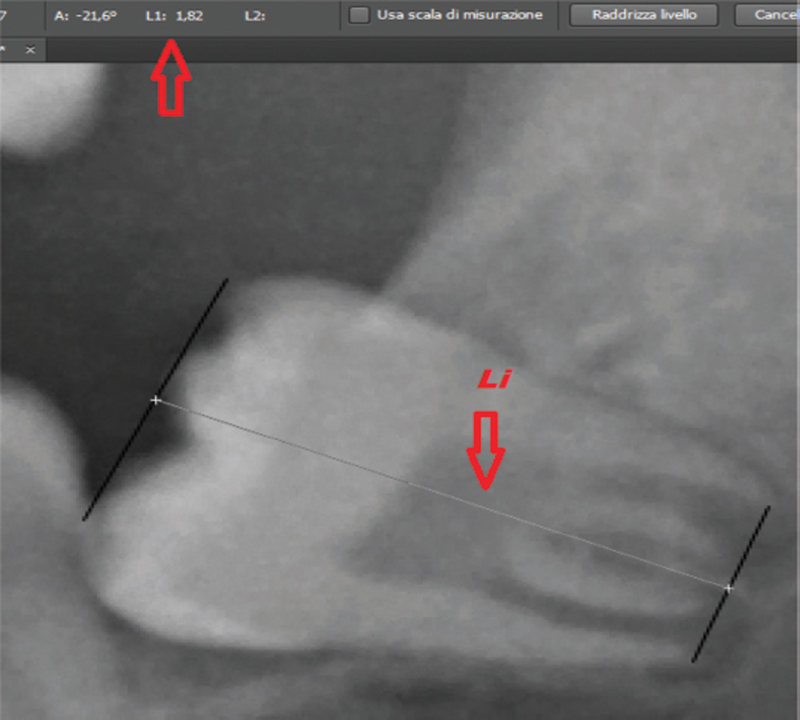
Radiographic measurements detail.
